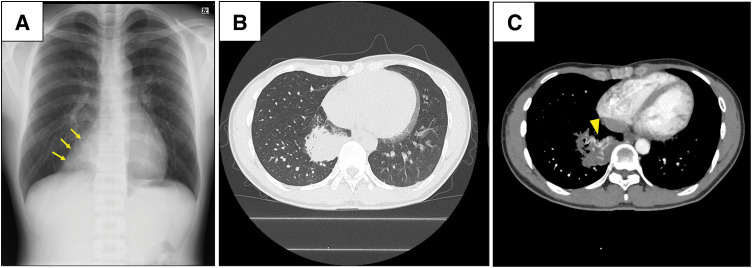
Preoperative imaging findings. (A) Chest radiograph obtained during a routine health check showing a well-circumscribed opacity in the medial aspect of the right lower lobe (arrows). (B) Contrast-enhanced CT demonstrating a mass in the right lower lobe. (C) Contrast-enhanced CT showing an enhanced vessel-like structure within the mass (arrowhead), suggesting a vascular lesion.
